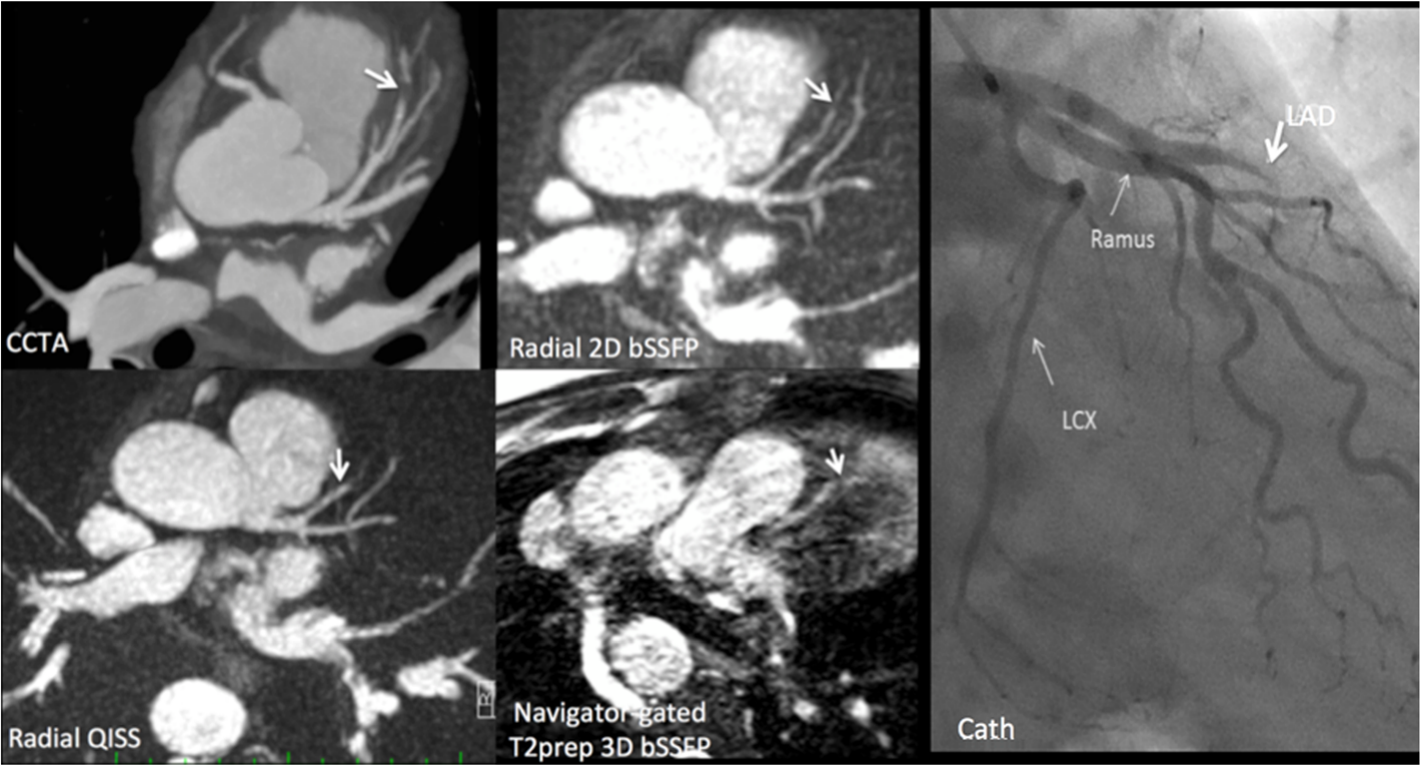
57-year-old patient with hyperlipidemia and chest pain. Breath-hold 2D T2-prepared bSSFP and free-breathing 3D T2-prepared bSSFP showed similar findings to the coronary CTA, which was prospectively interpreted as showing a severe LAD stenosis (arrow). However, radial QISS indicated an LAD occlusion, which was confirmed by subsequent x-ray coronary catheterization
